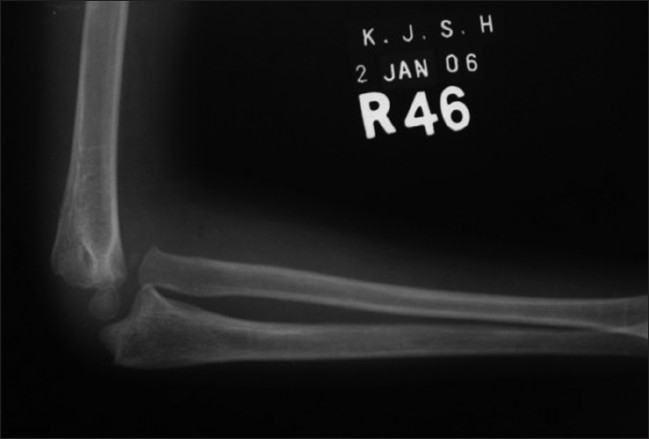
Lateral radiograph of elbow of 12 year old child showing missed Monteggia fracture
